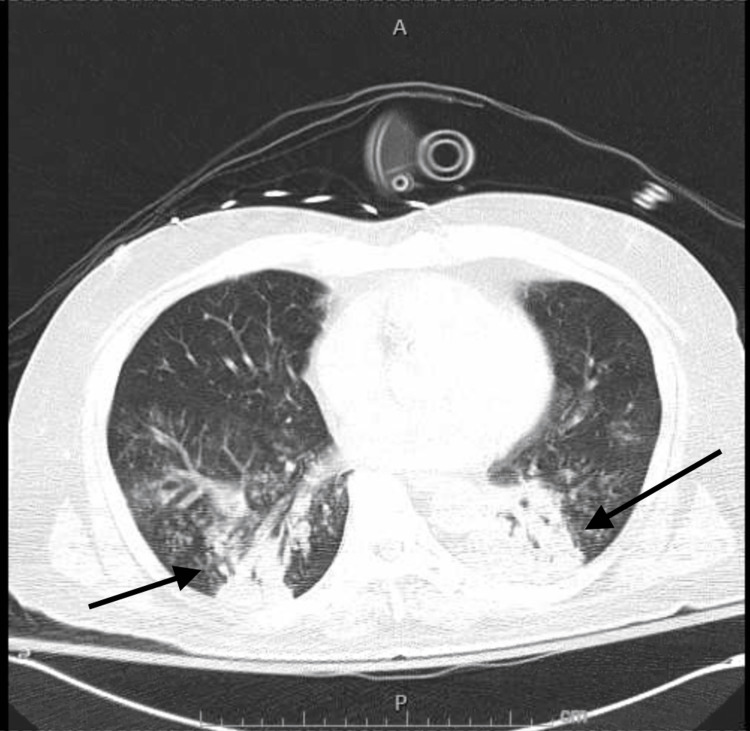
Computed tomography chest Computed tomography (CT) scan of the chest showing bilateral basal infiltrate (arrows).
